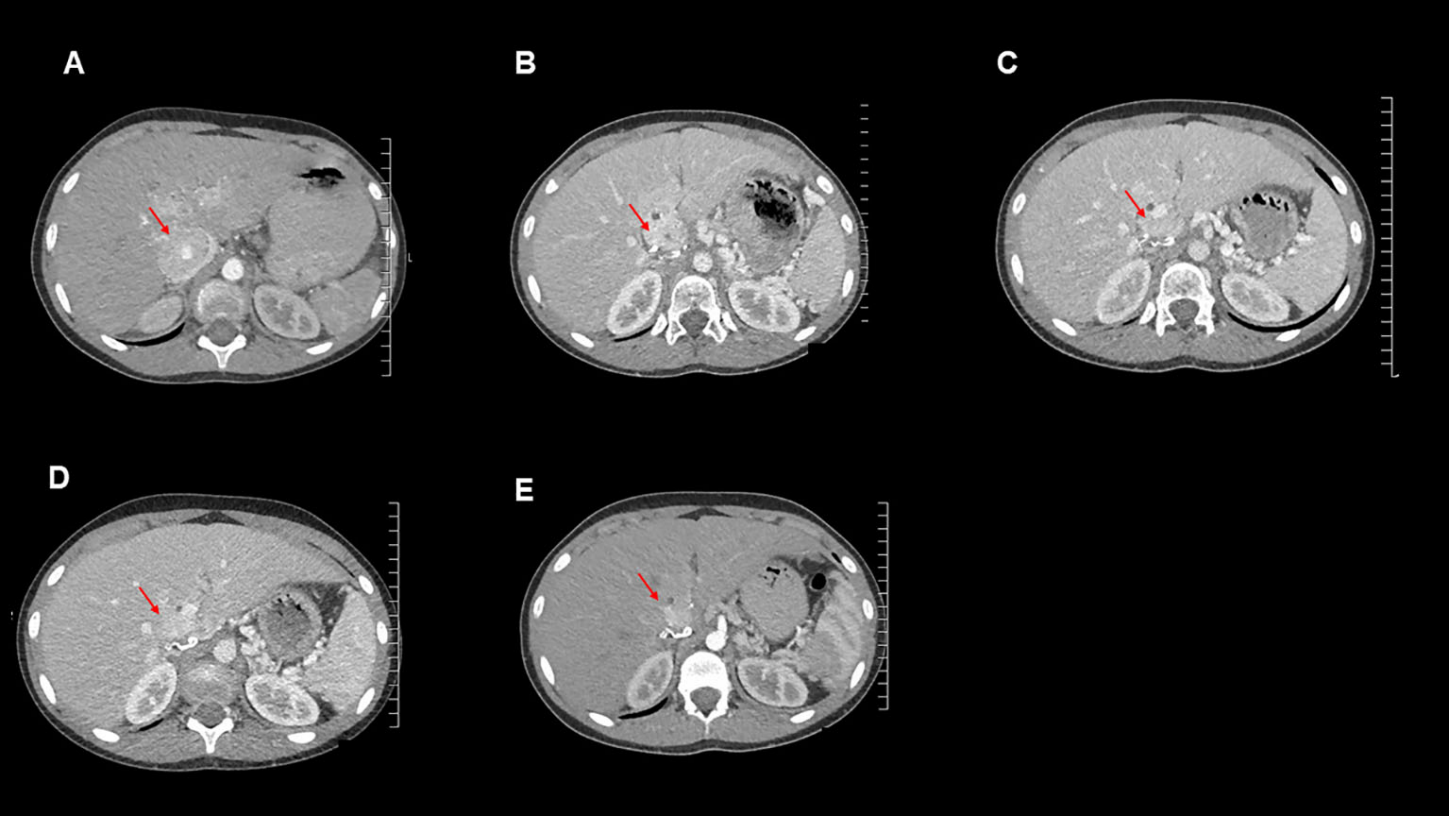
Axial contrast-enhanced CT images for this patient from the following time points: (A): September 2020 (post operation), (B): October 2021 (temozolomide therapy commenced), (C): January 2022, (D): April 2022, (E): July 2022.
